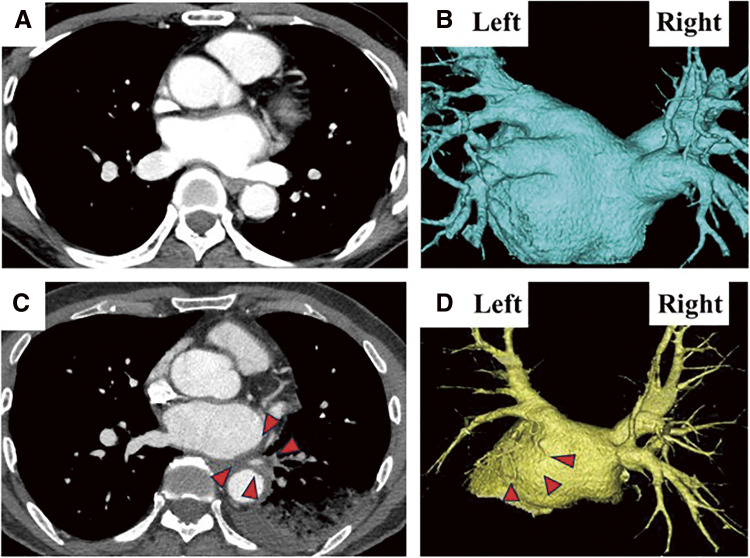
Pulmonary vein occlusion occurred after catheter ablation. (A and B) 2-dementional (2-D) axial images and 3-D construction images of contrast-enhanced chest CT scan before catheter ablation. (C and D) 2-D axial images and 3-D construction images of contrast-enhanced chest CT scan 6 months after catheter ablation.
